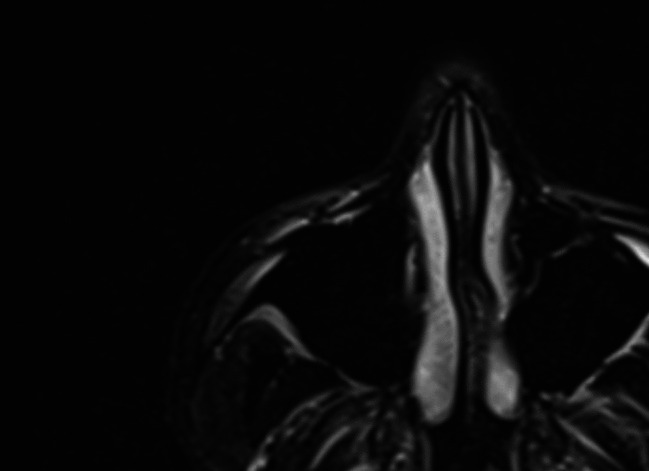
MRI of the head image. In the ventral central-left-side part of the medulla oblongata, in its middle section, an area of ​​increased signal with features of diffusion restriction (size 9 × 6 mm) corresponding to an ischemic focus is visible
